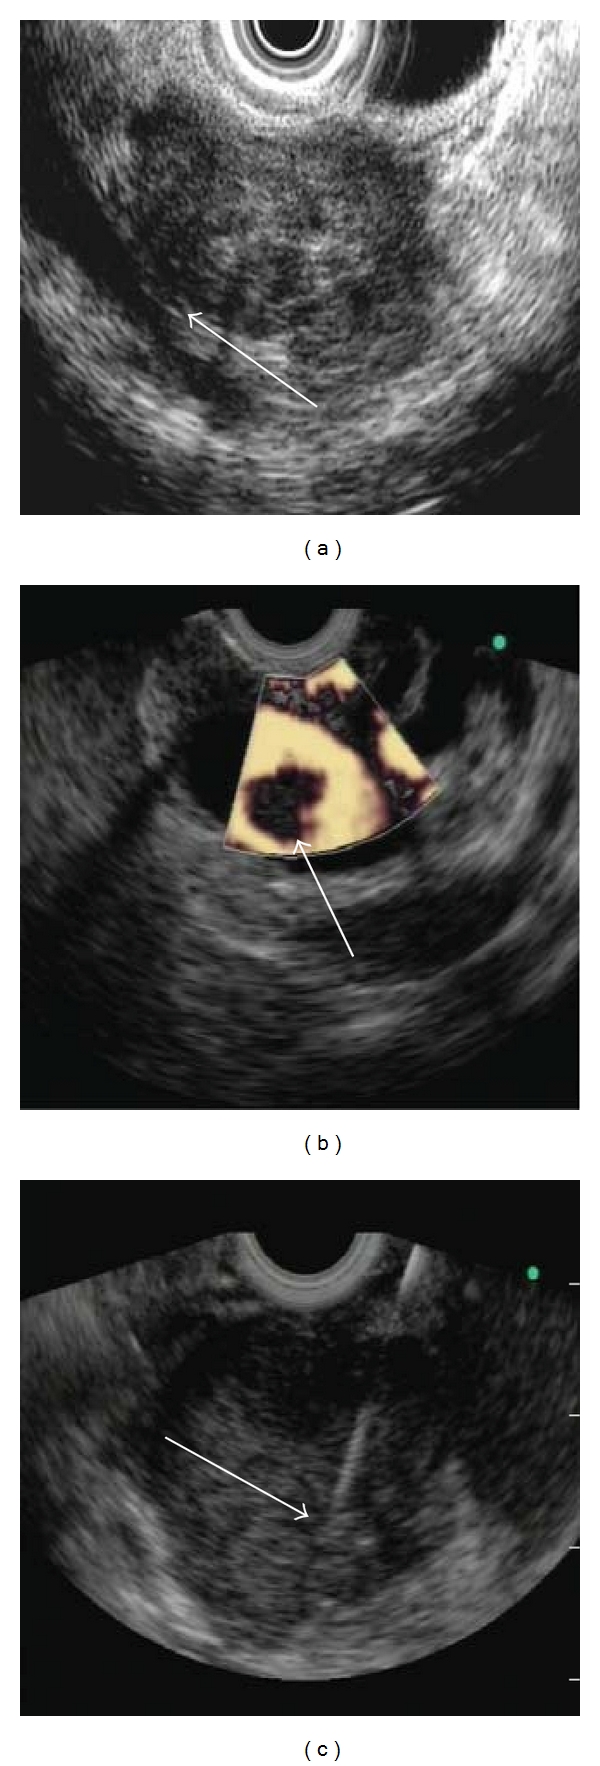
Images of endoscopic ultrasound in advanced pancreatic cancer: (a) tumor invading the vasculature, (b) portal vein thrombosis in advanced pancreatic cancer, (c) fine-needle aspiration biopsy of the pancreatic tumor.
